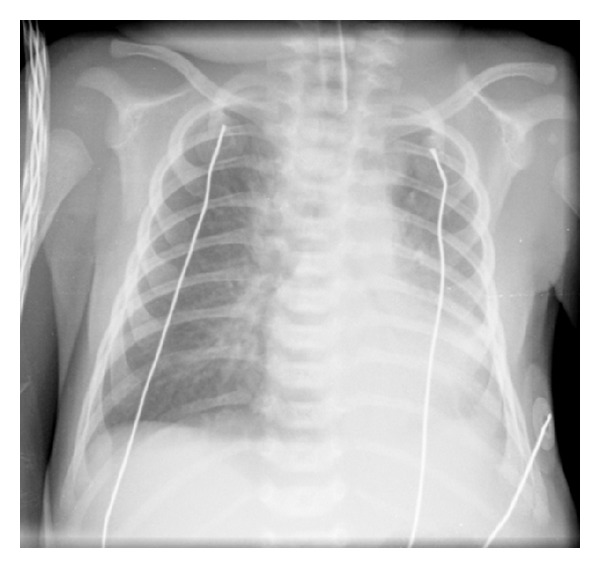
Consolidation pattern-chest radiograph showing pulmonary lower left lobe consolidation.
